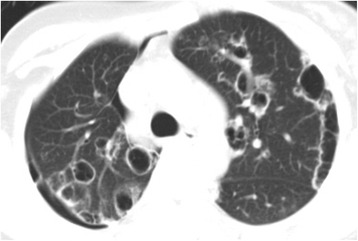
HRCT shows extensive cystic air spaces throughout both lungs with right pneumothorax
